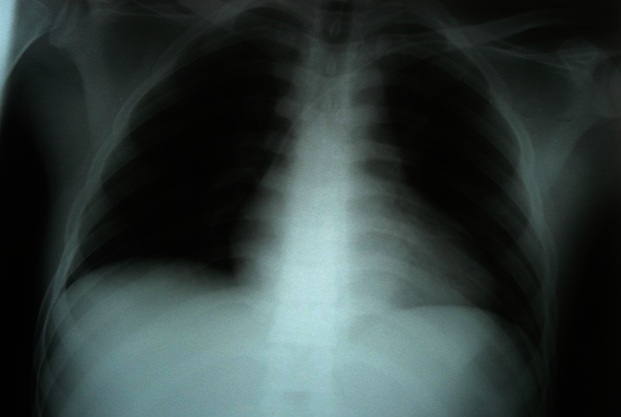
Radiographie du thorax chez un patient ayant présenté une intoxication massive à la cyperméthrine et à l'éthylène glycol. Elle montre la présence d'une silhouette cardiaque augmentée de taille témoignant de l'atteinte cardiaque toxique.
